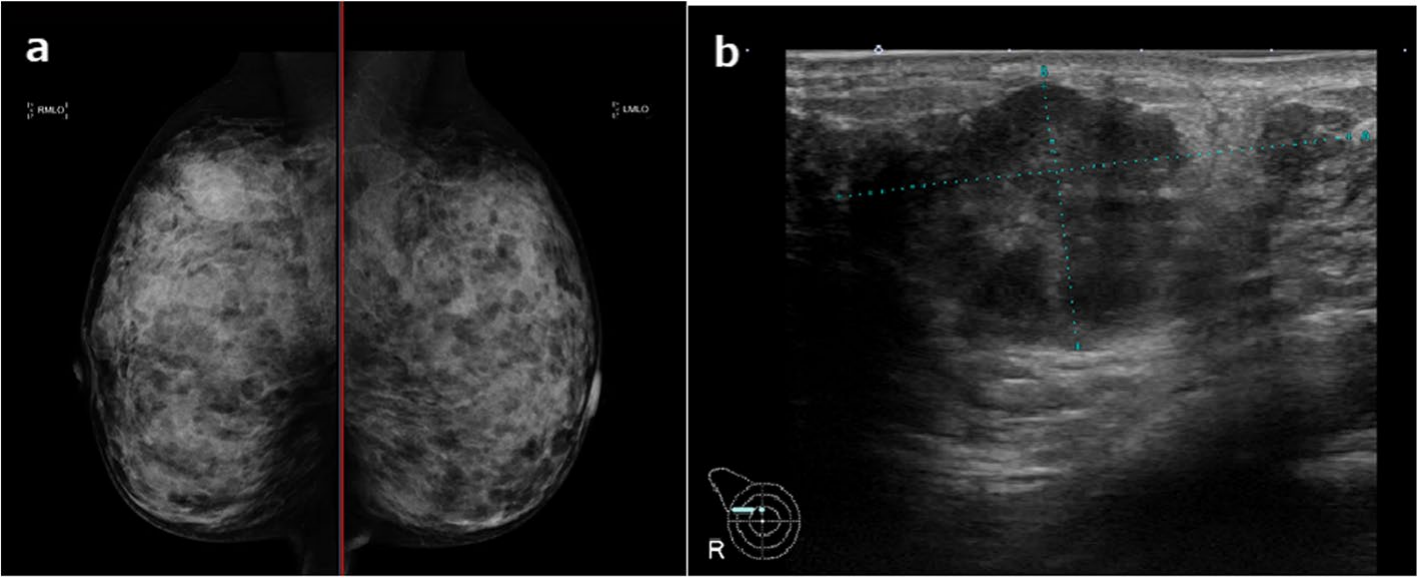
Mammography at diagnosis shows an indistinct high-density mass in the right breast (a). Ultrasonography identifies an irregular-shape low echoic mass in the right breast (b)
